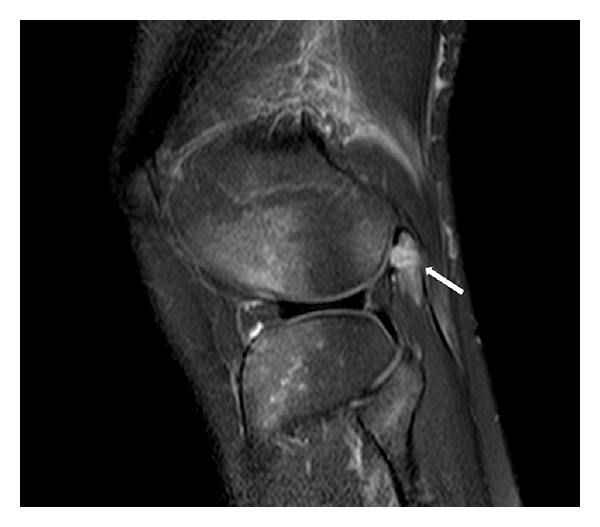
A sagittal T2-weighted fat-suppressed MRI demonstrated diffuse bone marrow edema pattern of the fabella (white arrow) associated with fracture seen on radiographs.
